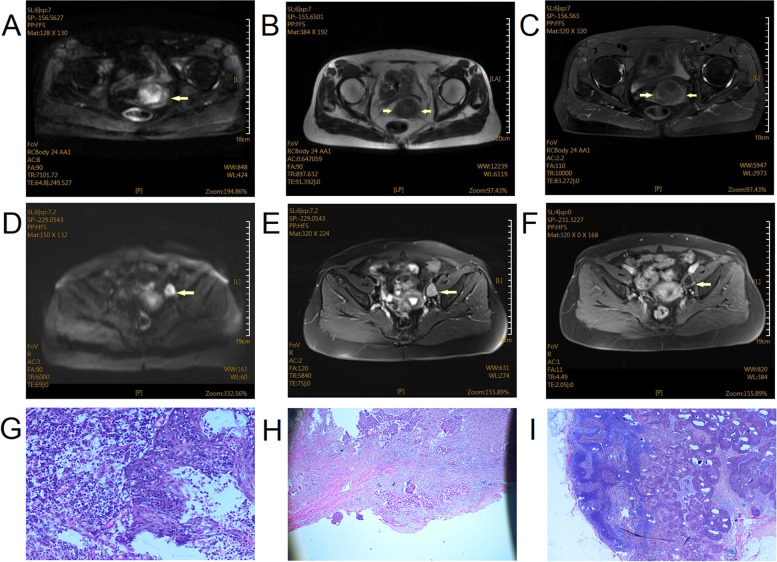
MRI imaging and pathological findings in patients with cervical cancer. A para-uterine infiltration DWI, B para-uterine infiltration T2WI, C para-uterine infiltration T2FS, D lymph node metastasis DWI, E lymph node metastasis T2FS, F lymph node metastasis enhancement, G cervical cancer pathology examination (uterine + double lateral attachment), H para-uterine infiltration pathology examination (uterus + double attachment), I lymph node metastasis pathology examination (uterus, bilateral fallopian tubes and left ovary)
